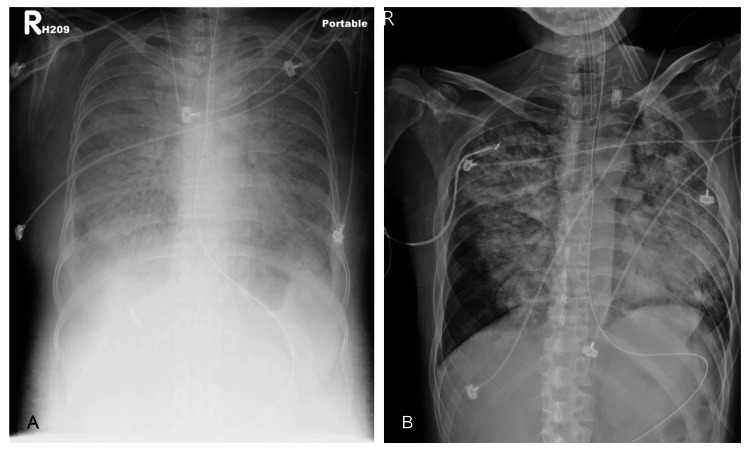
The chest radiographs in cases 1 (Figure 3A) and 2 (Figure 3B) showing severe diffuse bilateral coalescent opacities
